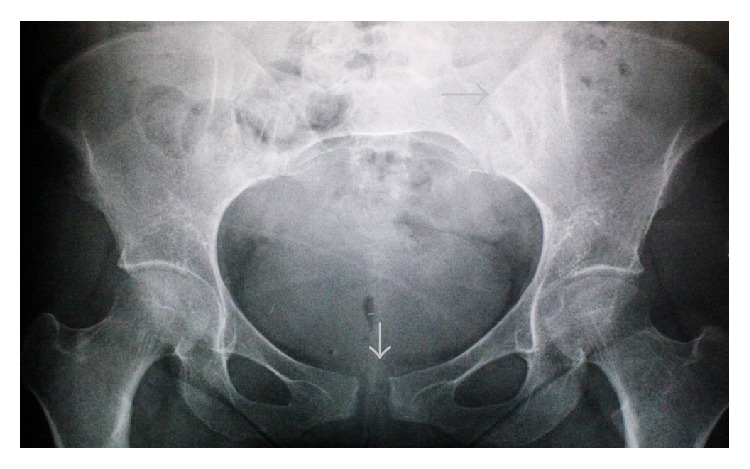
Anteroposterior radiograph of the pelvis demonstrating reduction of the pubic symphysis and left sacroiliac joint at 6 months postpartum.
